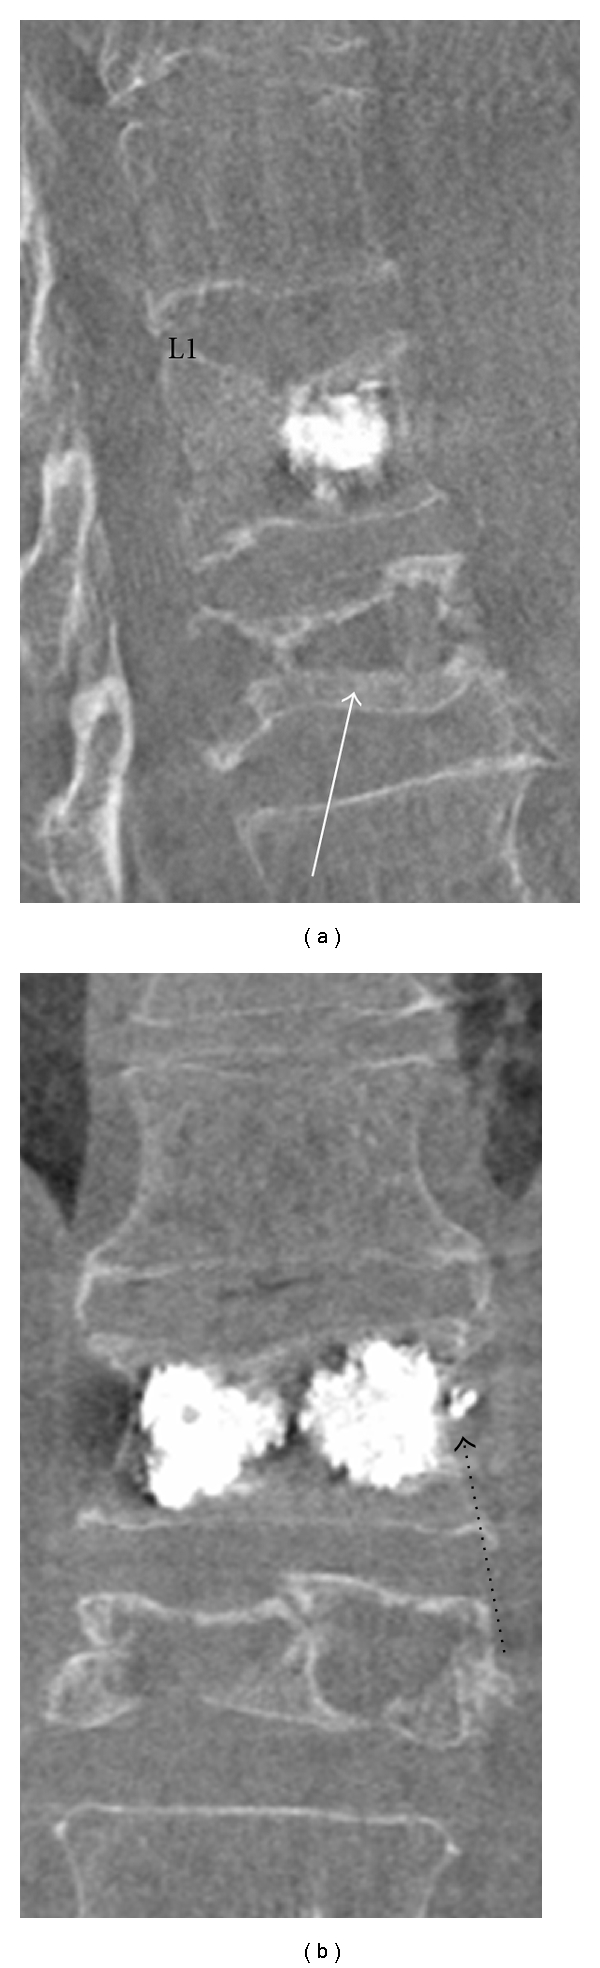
Modern biplane fluoroscopy allows on-table CT to be performed, with multiplanar reformats. Although the images acquired are susceptible to quantum mottle, the technique is invaluable in PV to quickly assess and confirm cement position and to assess for complications. Sagittal (a) and coronal (b) reconstructions (Siemens Axiom Artis biplane fluoroscopic unit) are shown in a 78-year-old man with recent VCF of L1 treated successfully with PV. An old VCF is noted at L2 (white arrow). A trace of lateral cement leak is noted to the left (dashed black arrow). This was asymptomatic.
